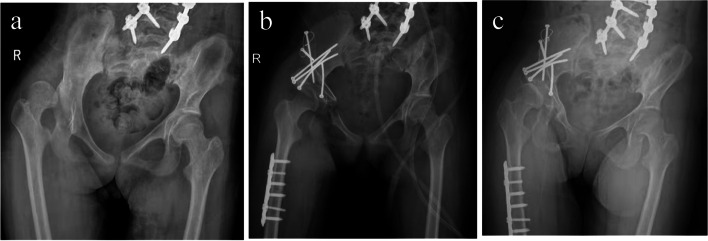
A 14-year-old female, quadriplegia. Pre-op GFMCS was V while post-op GFMCS was VI. Anteroposterior pelvic radiograph a before surgery; b immediately after PAO + DRO; c one year after surgery
